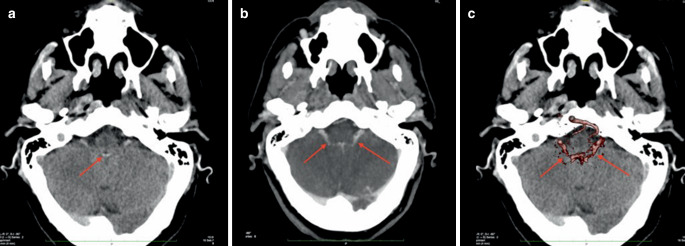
Example of a false-positive finding: the algorithm yielded a suspected intraventricular ICB. CT angiography shows that in this area of the suspected hemorrhage, choroid plexus vessels mimics a hemorrhage
